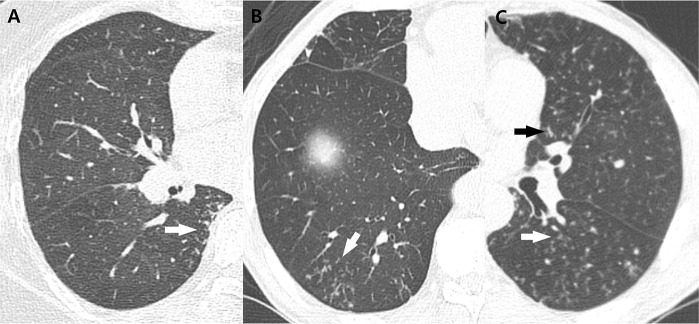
Examples of cellular bronchiolitis to CT scoring of MAC lung disease. (A) Mild cellular bronchiolitis in superior segment of right lower lobe of 67-year-old woman, scored as 1 point, manifesting as tree-in-bud patterns in the peripheral lung, 1 cm from the pleura (arrow). (B) Moderate cellular bronchiolitis in posterior basal segment of right lower lobe of 73-year-old man, scored as 2 points, showing centrilobular nodules or tree-in-bud patterns involved greater than 1–3 cm from the pleura (arrow). (C) Severe cellular bronchiolitis in superior segment of left lower lobe and lingular segment of left upper lobe of 59-year-old man, scored as 3 points, showing centrilobular nodules or tree-in-bud patterns extending to the central lung (arrows).
